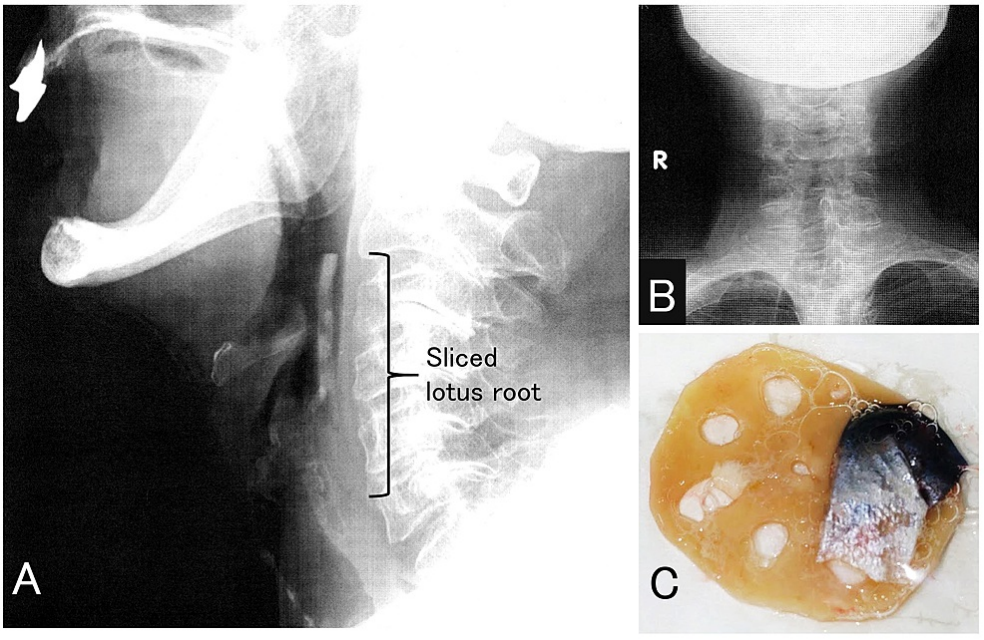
X-ray images and removed lotus root A: Side view of the neck (x-ray). The sliced lotus root was present in the hypopharynx, and it wedged into the proximal part of the esophagus. B: Frontal view of the neck (x-ray). C: The removed lotus root and fish skin. The diameter and thickness of the lotus root were 61 mm and 4 mm, respectively.
